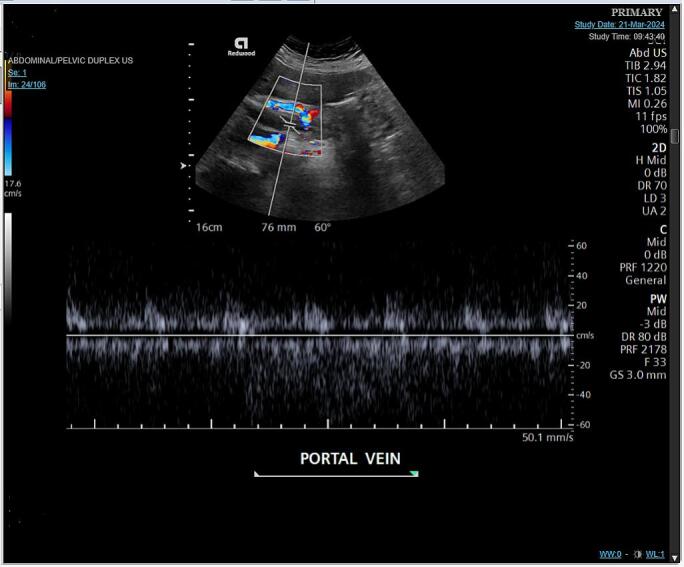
Ultrasound abdomen and pelvis Doppler venous duplex showed thrombosed portal vein.
